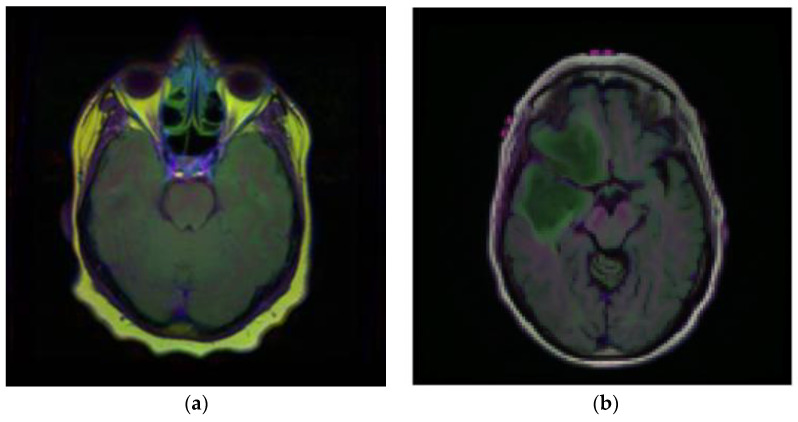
Samples of Brain MRI Images (a) Normal Image, (b) Tumor Image.
